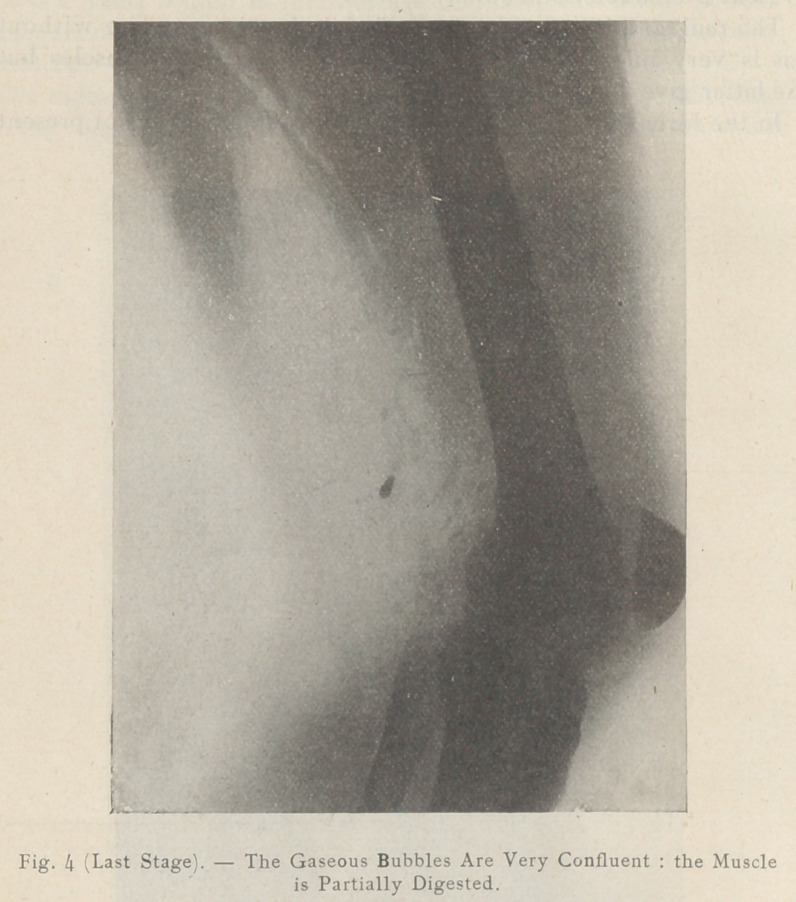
# Radiographic Aspects of Gangrenous Infections of War Wounds and of Gas Gangrene in Particular
